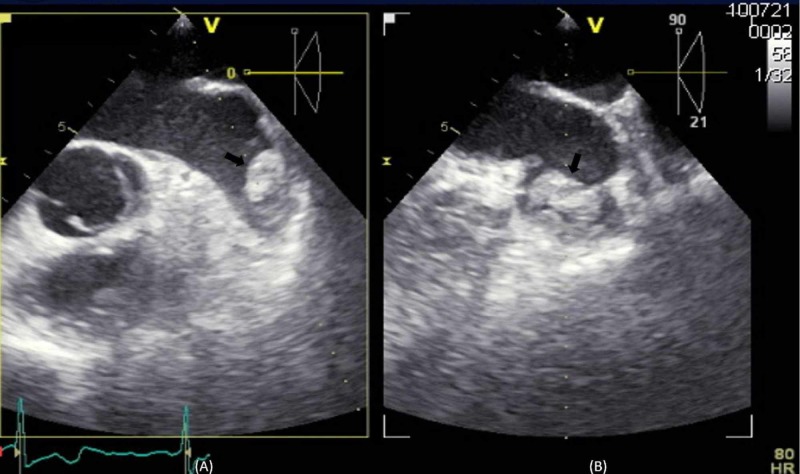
Trans-esophageal echocardiogram biplane view of the left atrial appendage. Demonstrating the mass (black arrow) concerning for thrombus in transverse (A) and longitudinal axis (B) in the left atrial appendage.
